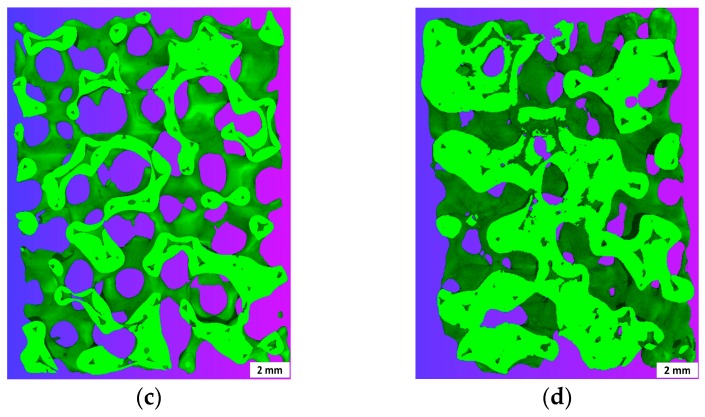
µ-CT 3D reconstruction of 3-mm thick slices from ZTA foams after recoating: (a) ZTA20-80; (b) ZTA20-R1; (c) ZTA20-R2 and (d) ZTA20-R4.
